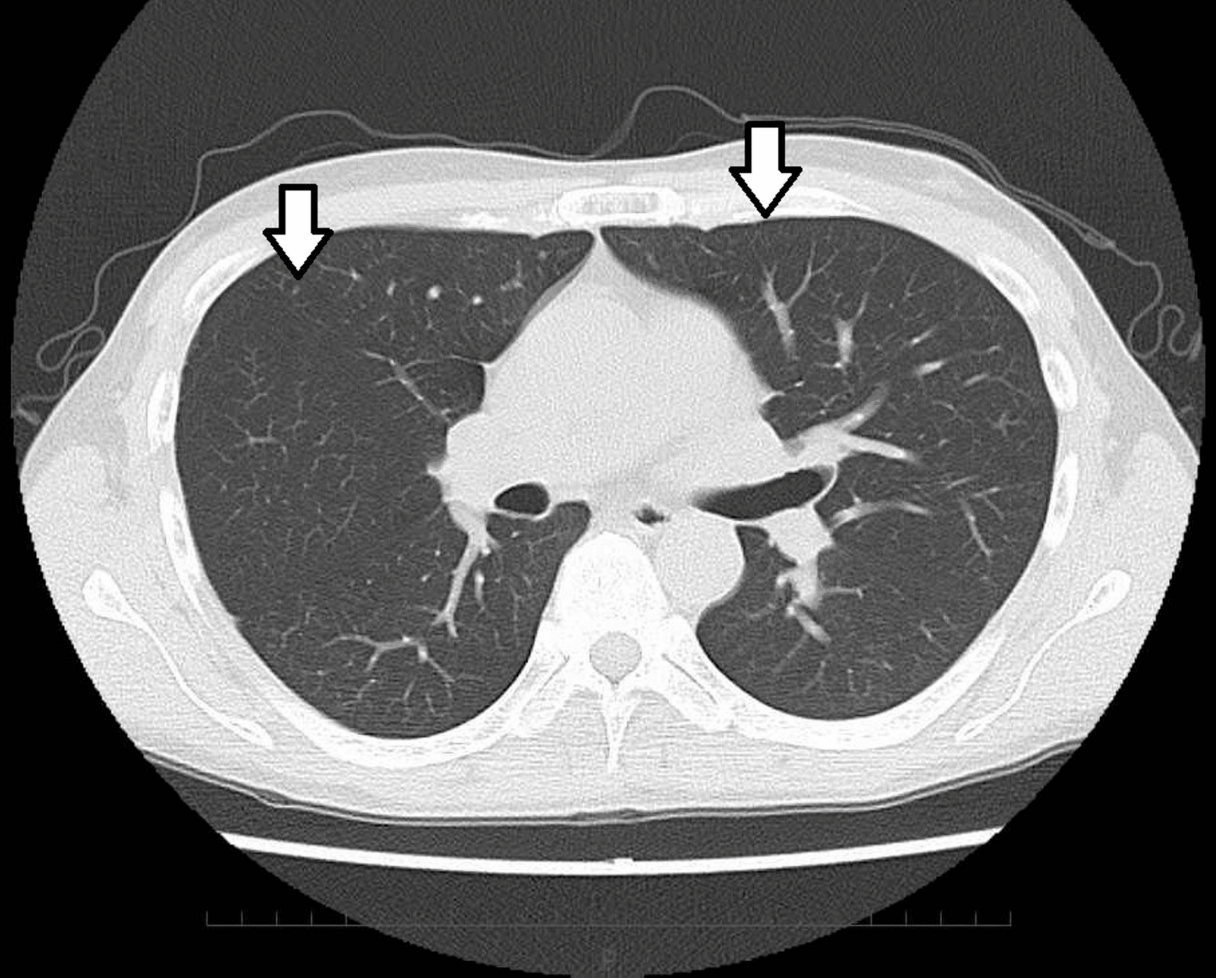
CT scan at follow-up two years after initiation of EV showing complete response with the resolution of all lesions (white arrows). EV: enfortumab vedotin
